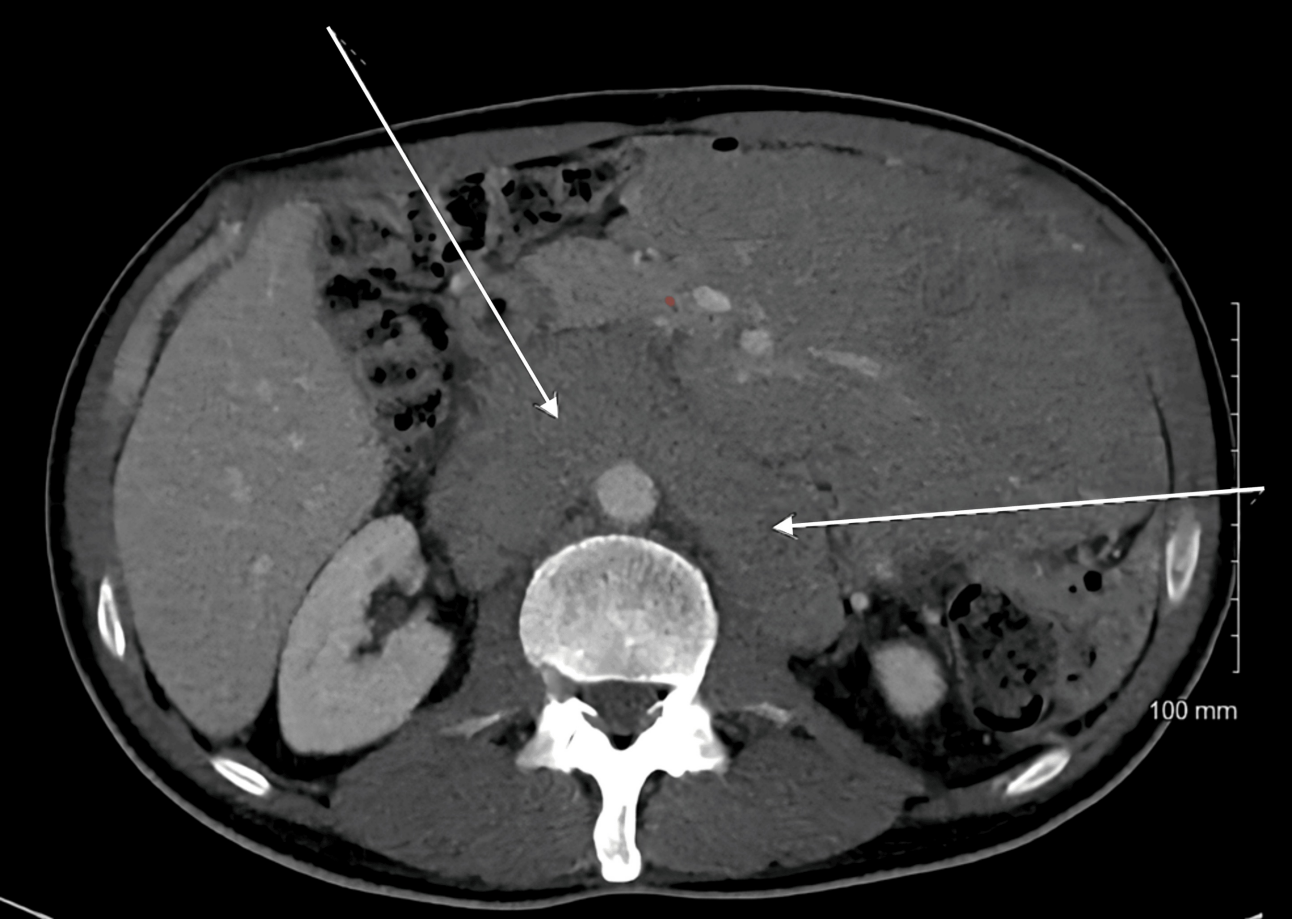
CT abdomen showing massive retroperitoneal and mesenteric adenopathy (white arrows)
